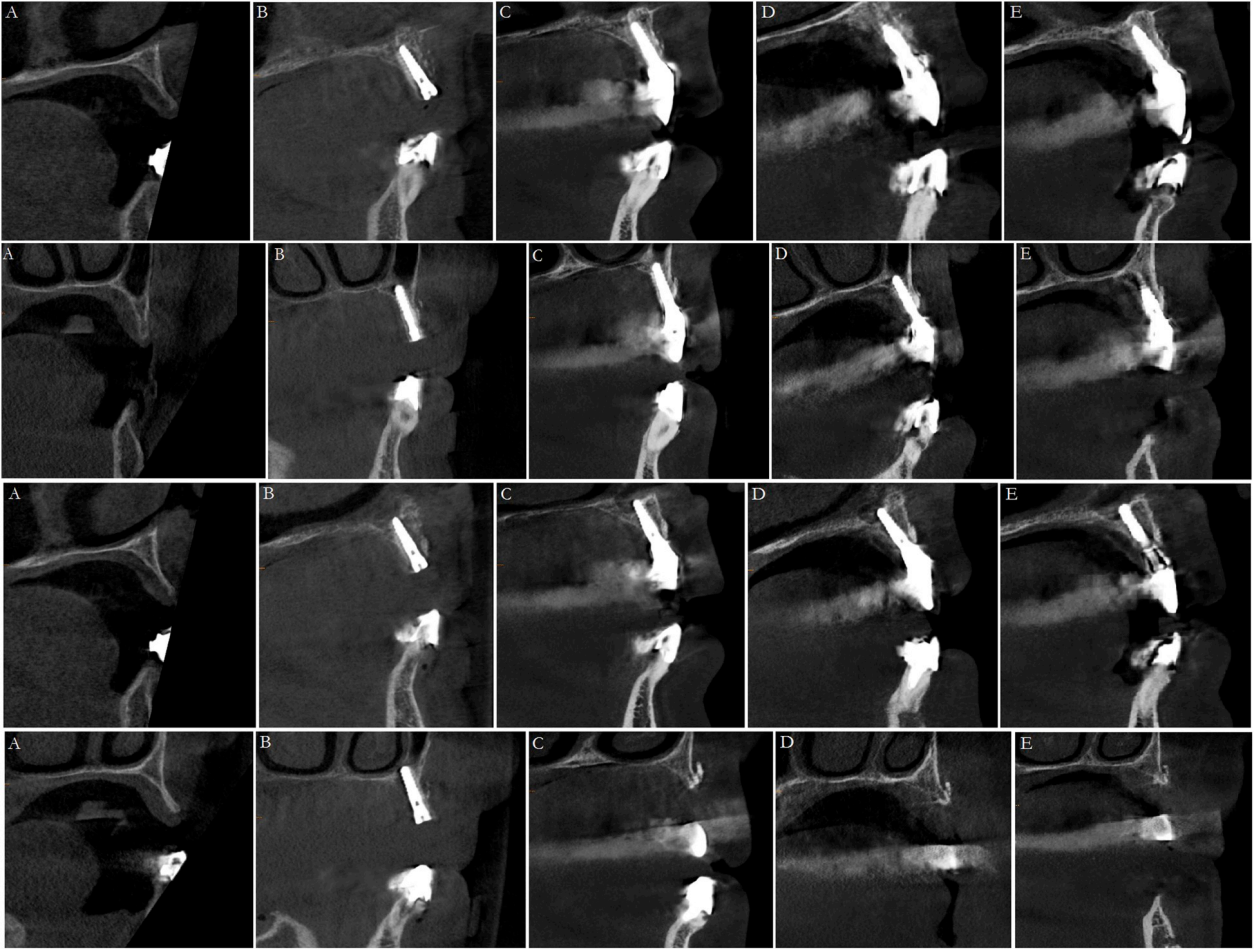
A-E,* Cone beam computed tomographic (CBCT) imaging, comparing alveolar ridge with before and after placing of implants in region 12, 14, 22, 24 *A,* Pre-surgical status revealing moderately deficient alveolar ridge dimension of the maxillary ridge 2015. *B,* CBCT after surgery using ARST and dental implant placement; note that the alveolar ridge was sufficiently expanded. *C,* CBCT 2017. *D,* CBCT 2018. *E,* CBCT 2019.
